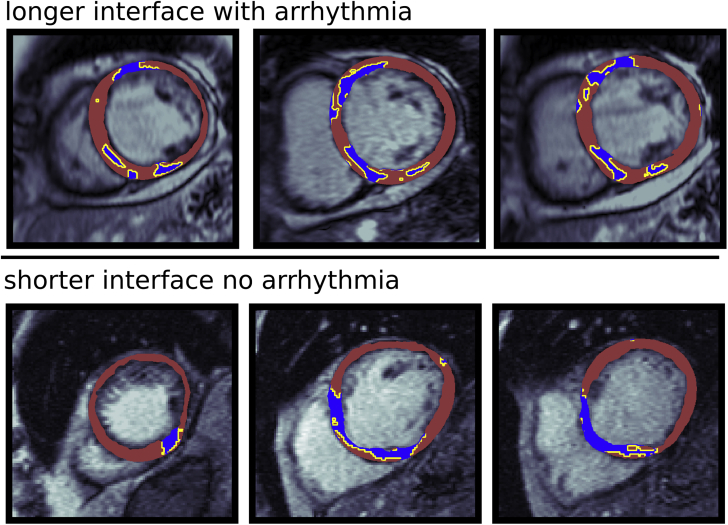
Example LGE-MRI Slices From 2 Patients With Similar LGE Volumes but Differing Interface Areas and Outcomes The LGE volumes of the 2 patients are similar (top: 14.5 cm^3^, bottom: 14.0 cm^3^), but the interface areas (top: 156.3 cm^2^, bottom: 86.8 cm^2^) and outcomes (top reached arrhythmic endpoint; bottom did not) differ. LGE = late gadolinium enhancement; MRI = magnetic resonance imaging.
